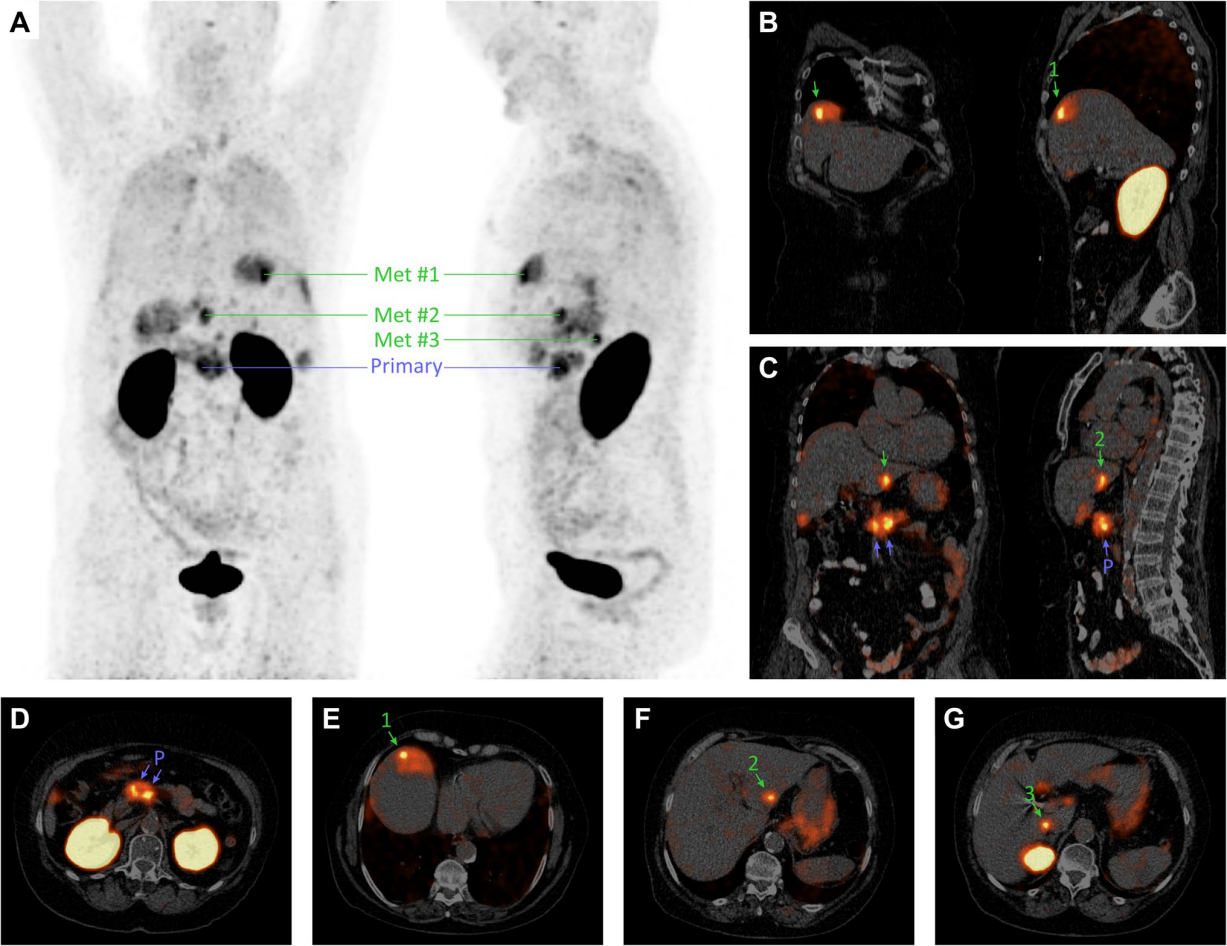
^68^ Ga-Trivehexin PET/CT of metastatic PDAC (confirmed by biopsy) in patient #4 (f, 80 y, 80 kg, 105 MBq, 120 min p.i.). PET is scaled to SUV 10 in all images. A Anterior and sagittal MIP, showing ^68^ Ga-Trivehexin uptake in the primary tumor (SUV_max_ = 9.8–10.9) and in 7 metastases. Selected metastases highlighted in panels B–G are indicated (SUV_max_ of #1, #2, and #3 are 10.9, 10.0, and 9.9, respectively). B Coronal and sagittal PET/CT slices through metastasis #1. C Coronal and sagittal PET/CT slices through primaries and metastasis #2. D, E, F, G, axial PET/CT slices though primaries and metastases #1, #2, and #3, respectively
